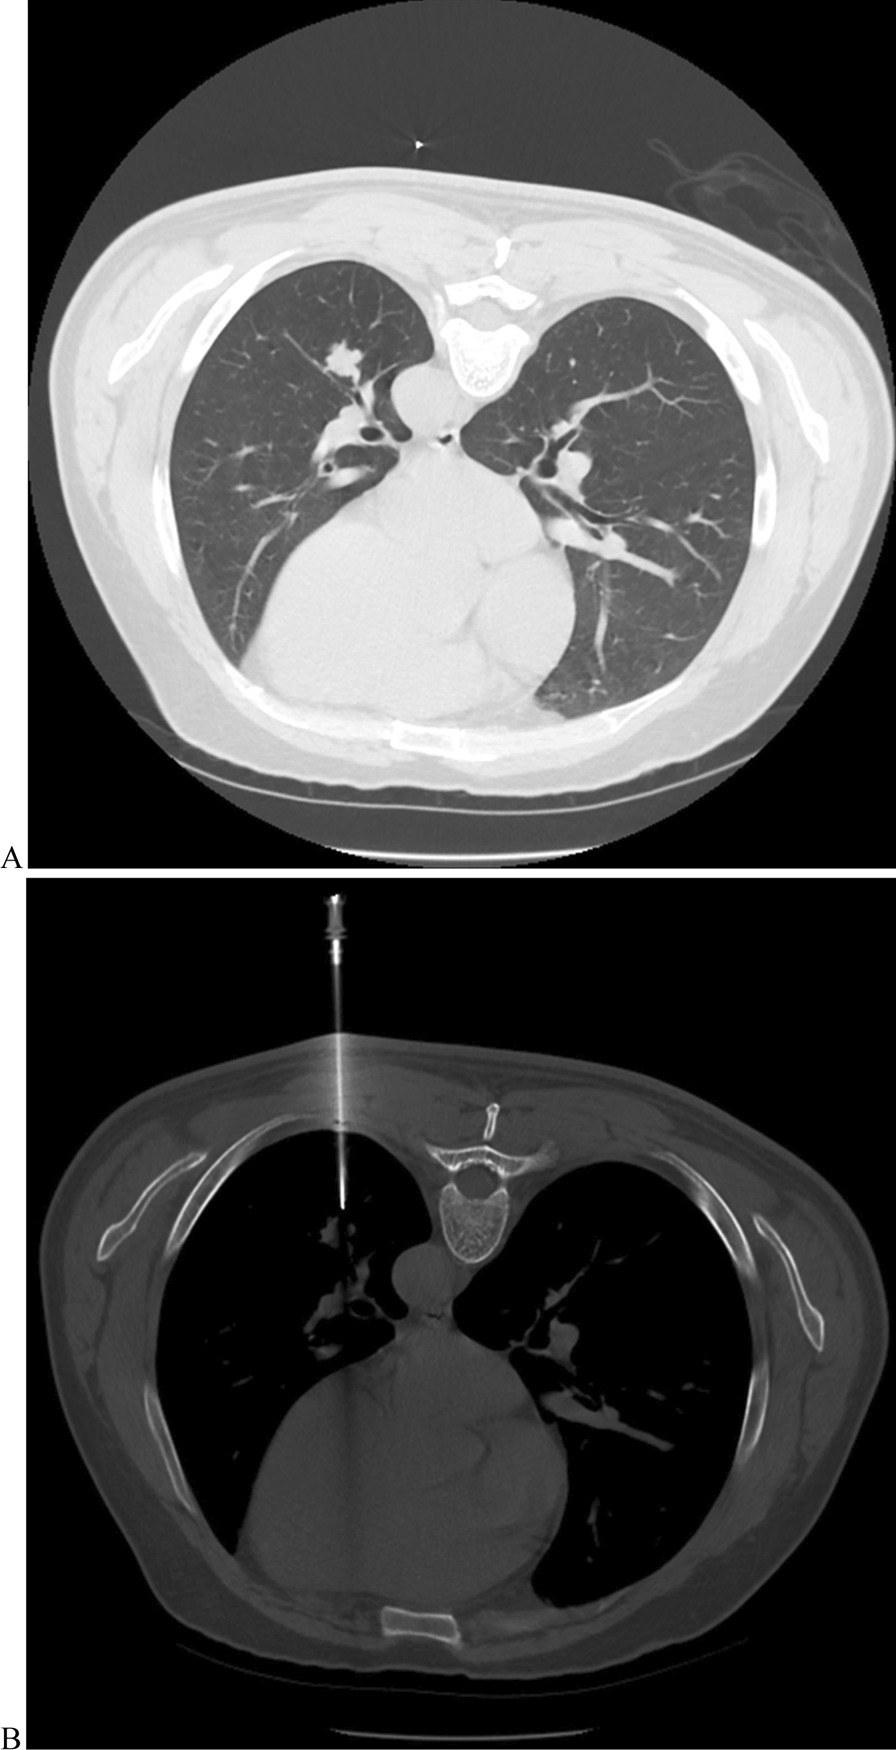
The images for standard-dose CT-guided biopsy for PN. a Preoperative CT for the PN; b the procedure of standard-dose CT-guided biopsy
